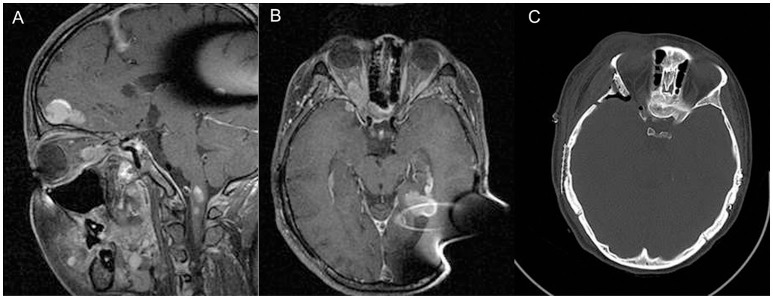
Sagittal (A) and axial (B) contrast enhanced T1-weighted MRI of patient D, demonstrating right orbital apex meningioma. Panel (C) shows post-operative CT demonstrating extent of bony decompression of lateral orbit.
